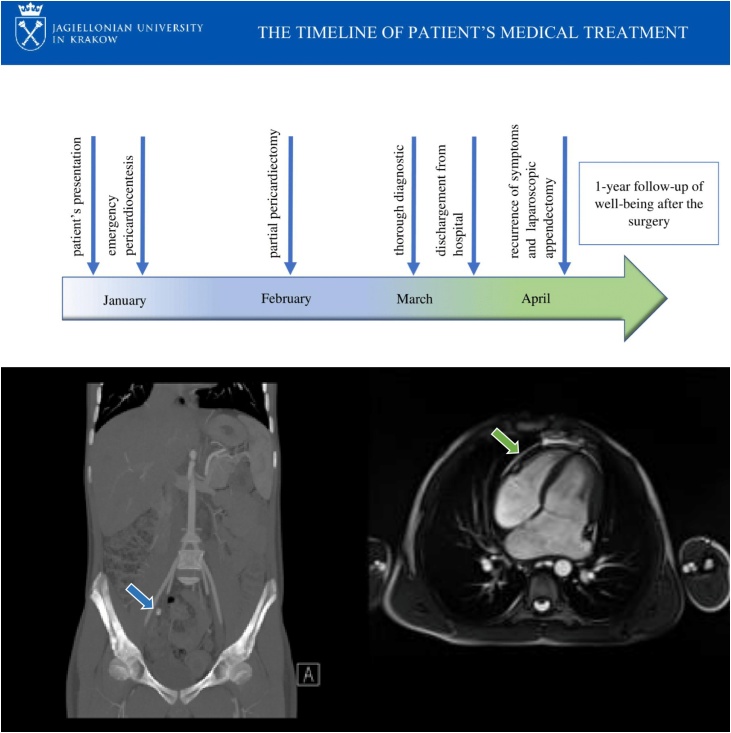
On the top the time-line of patient’s history. On the left: the contrast-enhanced computed tomogram revealed bilateral pleural effusions, fluid in peritoneal cavity and prominent appendix with surrounding inflammatory changes containing an appendicolith of size 19 mm x 12 mm (the blue arrow). On the right: heart magnetic resonance imaging with intravenous contrast medium showing a process in the pericardium (the green arrow).
